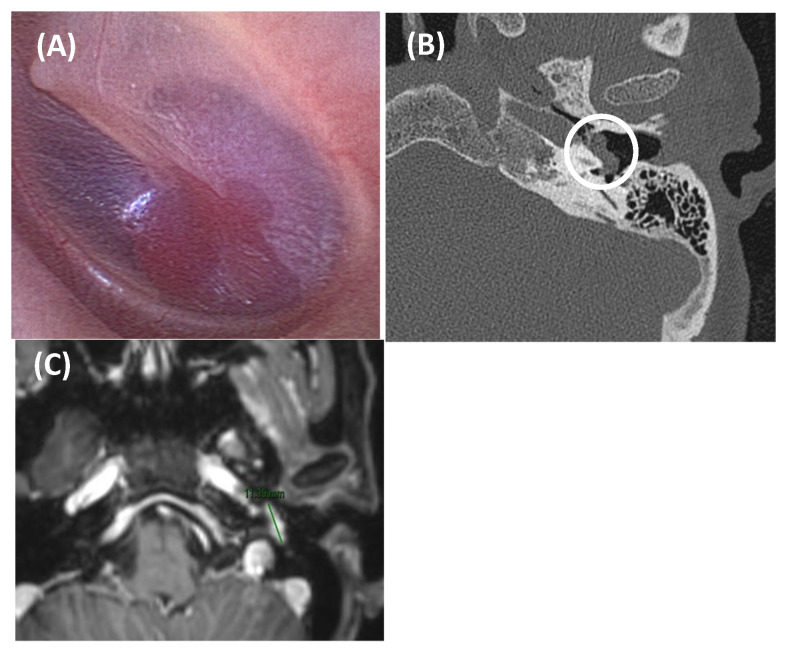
Tympanic paraganglioma in an 81-year-old woman presented with somatosound in the left ear, displaying arterial characteristics. Otoscopy revealed a red retrotympanic mass (image (A)). An axial section of a CT scan (Image (B)) showed a small soft tissue tumor in the left ear over the cochlear promontory (outlined by a white circle), about 8.3 mm in anteroposterior diameter, suggestive of tympanic paraganglioma. Axial post gadolinium T1-weighted MRI scan (Image (C)) confirmed a mass at this level with intense gadolinium enhancement (green line). In this case, due to the patient’s age and associated risks, a decision was made to monitor the condition with regular follow-up.
